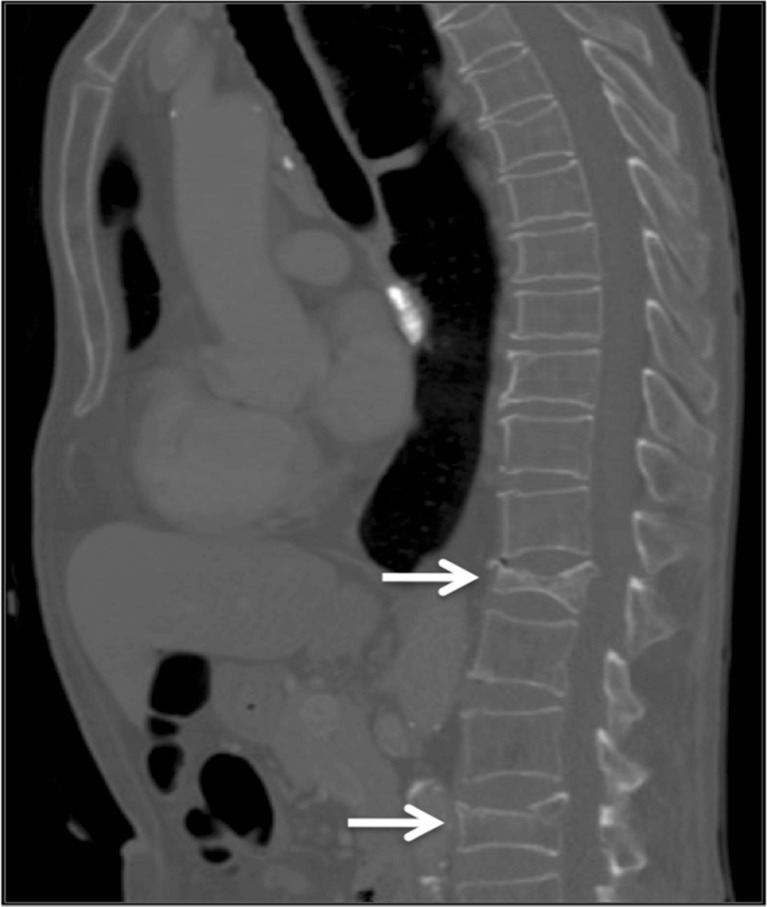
A sagittal section from a CT scan of a patient aged 71 years. The grade 3 L1 fracture and grade 2 L4 fracture (indicated) were not reported by the radiologist. The patient went on to suffer a hip fracture 3 years later
